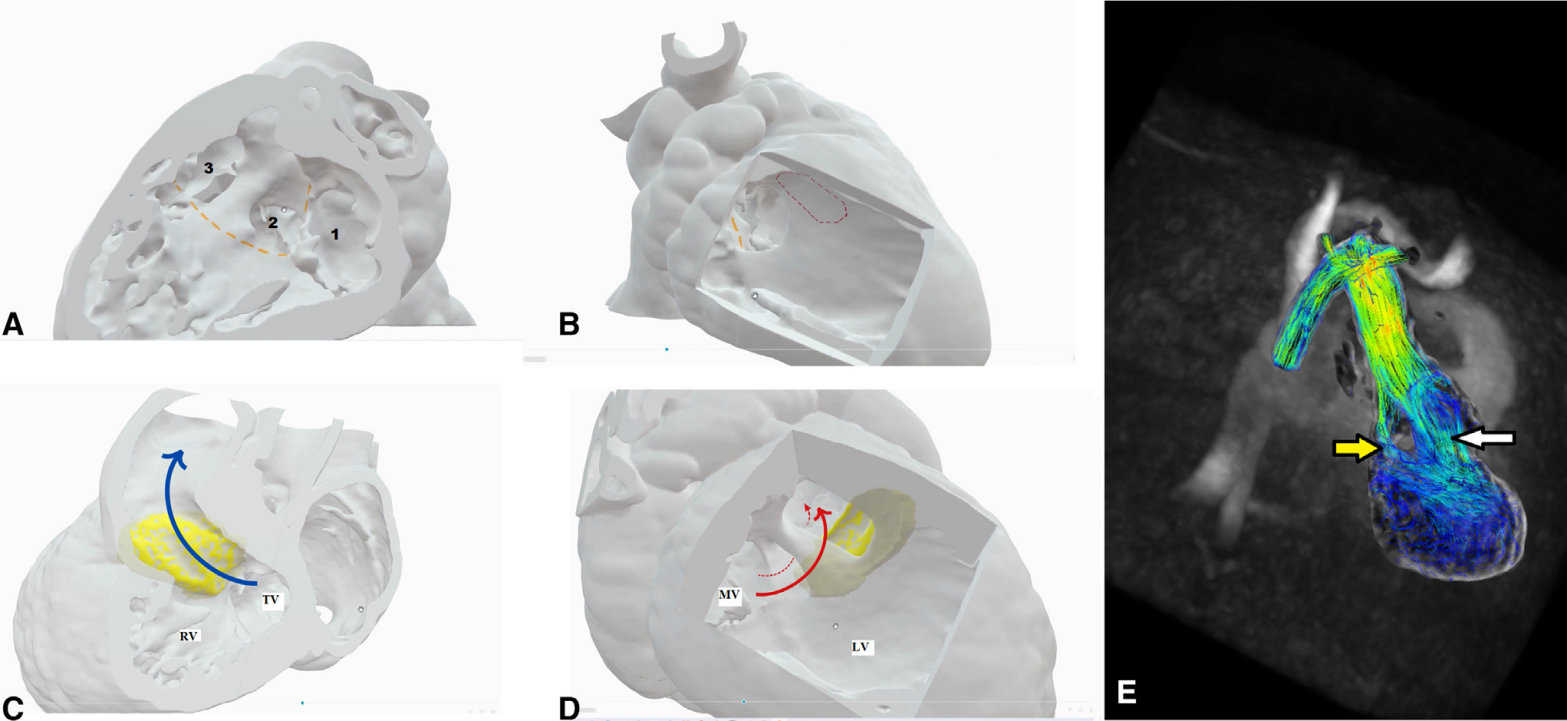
Preoperative modeling permitted us to anticipate the site of a new interventricular communication, which would open to the subpulmonary outlet tract. The panels show its site as seen from the *right* (A) and *left* (B) ventricles, along with the planned suture line (*yellow lines*), where 1 indicates the tricuspid valve, 2 indicates the original interventricular communication, and 3 indicates the new interventricular communication (marked in *red* in B). Postoperative modeling shows the subsequent anatomy and the flow from both interventricular communications to the aorta, again as seen from the *right* (C) and the *left* (D) ventricles. E, Magnetic resonance imaging permitting 4-dimensional analysis of flow. Of the flow, three-quarters was through the new interventricular communication (*white arrow*), rather than the original defect (*yellow arrow*). *RV*, Right ventricle; *TV*, tricuspid valve; *MV*, mitral valve; *LV*, left ventricle.
